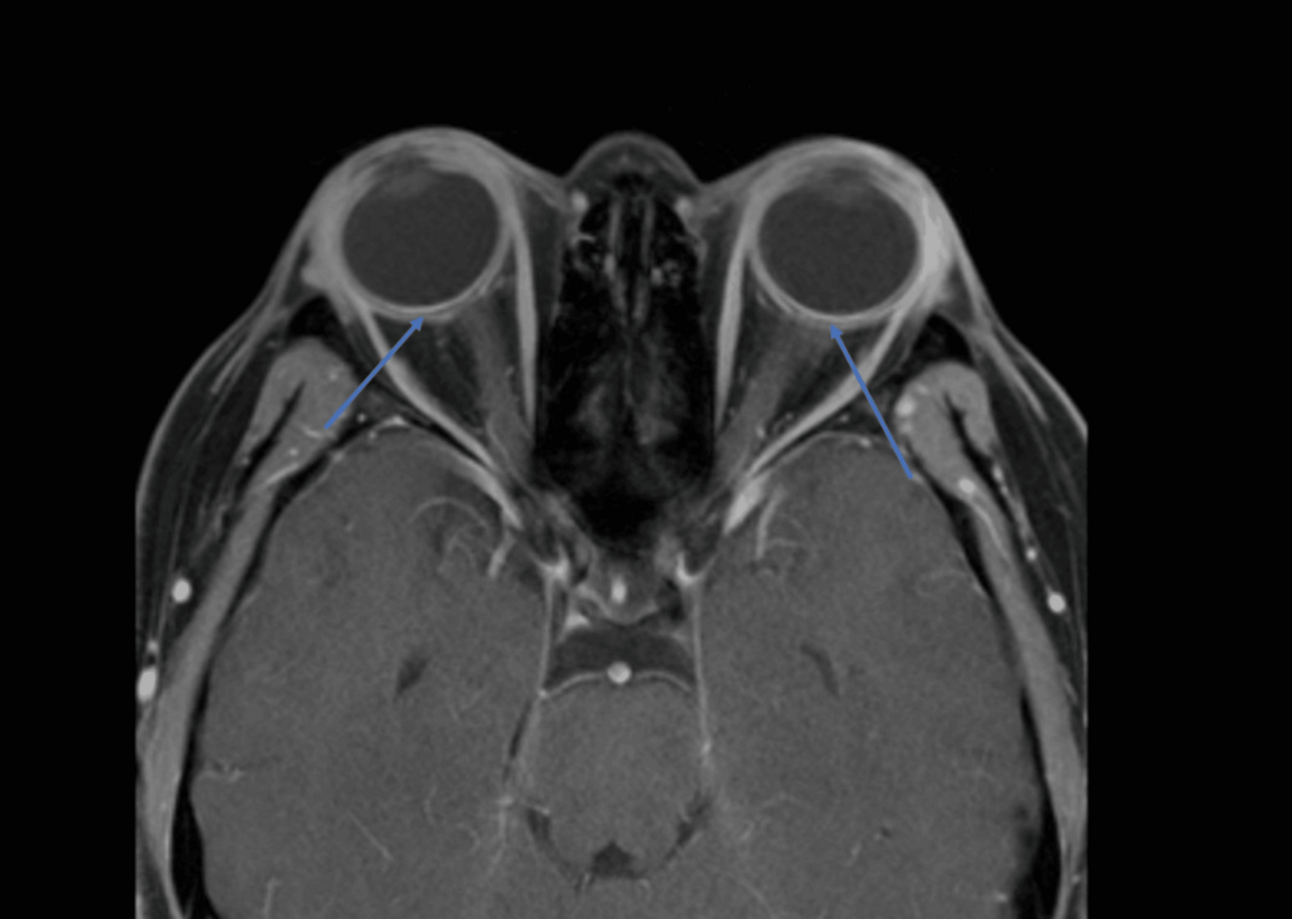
Orbital MRI demonstrating bilateral scleral thickening and enhancement without discrete mass
